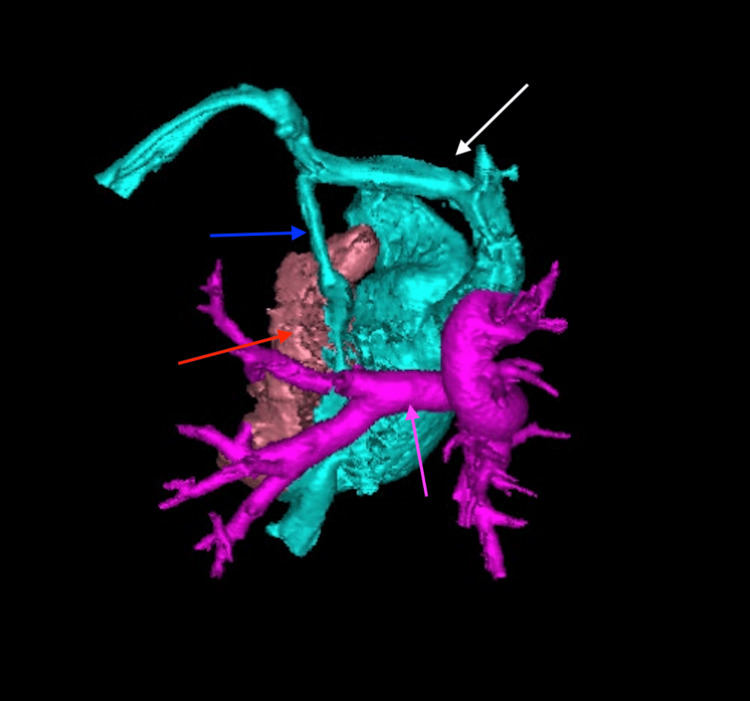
Three-dimensional computed tomography angiography reconstruction (posterior view) showing bilateral superior vena cavae: right superior vena cava (white arrow) and persistent left superior vena cava (blue arrow) The pulmonary venous confluence is indicated by the red arrow. The main pulmonary artery (pink arrow) arises from the right ventricle.
